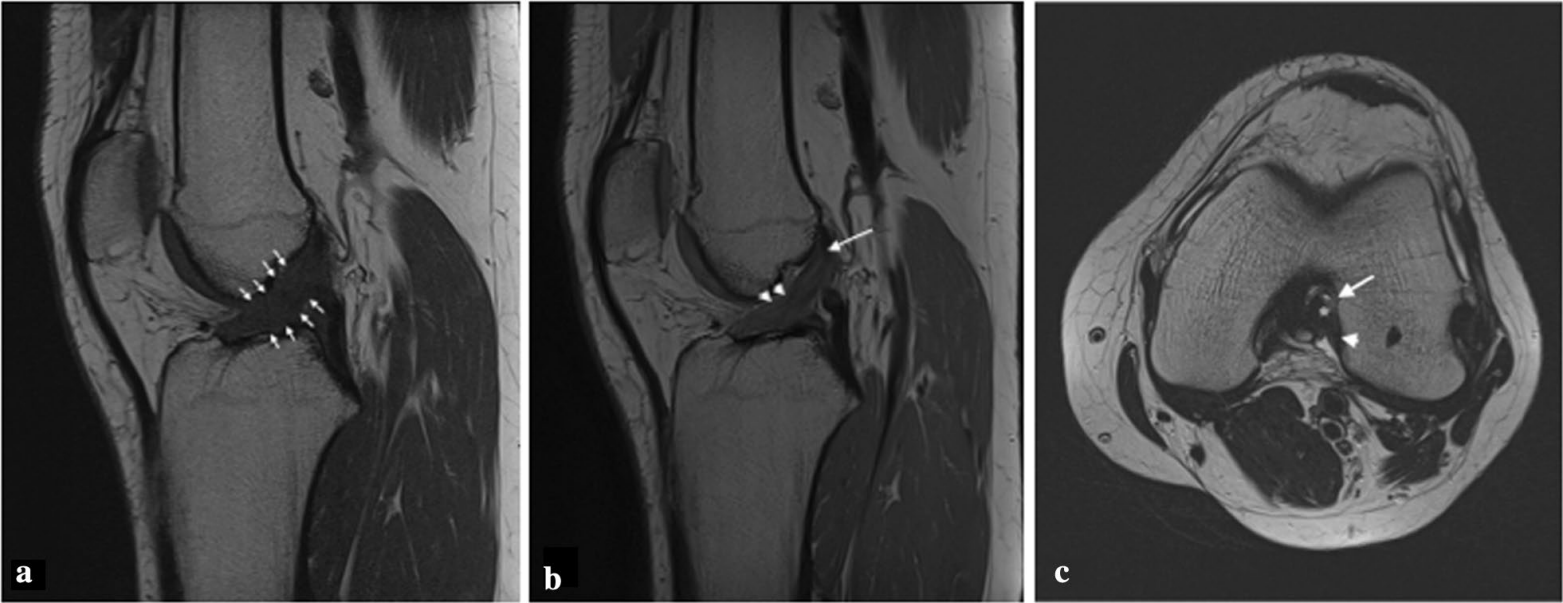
a Sagittal T1-weighted MR image revealing diffuse thickening, poor definition of anterior edge of ACL (arrows) with increased signal intensity within the ACL substance; b 3D PD-weighted, sagittal multiplanar reformatted (MPR) image showing abnormal intrasubstance high signal intensity of middle and distal third of ACL (arrowheads) and well-discernible fibers in proximal region (arrow); c axial T1-weighted MRI showing focal fluid signal along the midsubstance of ACL (arrow) in the intercondylar notch. Posterior fibers of ACL remain attached to femur (arrowhead), while anterior fibers seem to wear thin and detach (asterisk)
